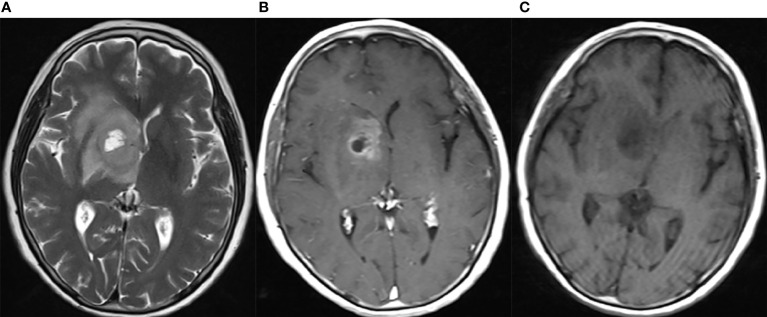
Neuroradiological findings for eGBM case 11. Neuroradiological results for eGBM case 11. (A) A heterogeneous lesion with necrosis and perilesional oedema on T1 in the right basal ganglia with significant midline shift, 23 mm × 28 mm × 17 mm in size. (B) A heterogeneous lesion with perilesional oedema (T2). (C) A rim-enhancing mass with perilesional oedema (T1-weighted enhanced).
